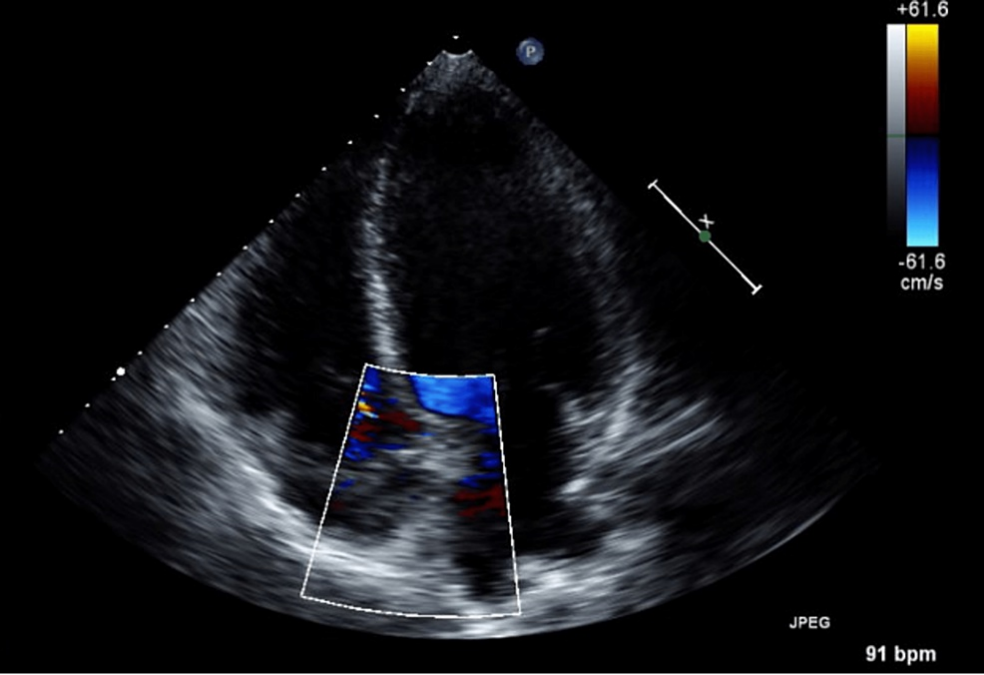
Transthoracic color Doppler echocardiography showing flow across suspected patent foramen ovale
